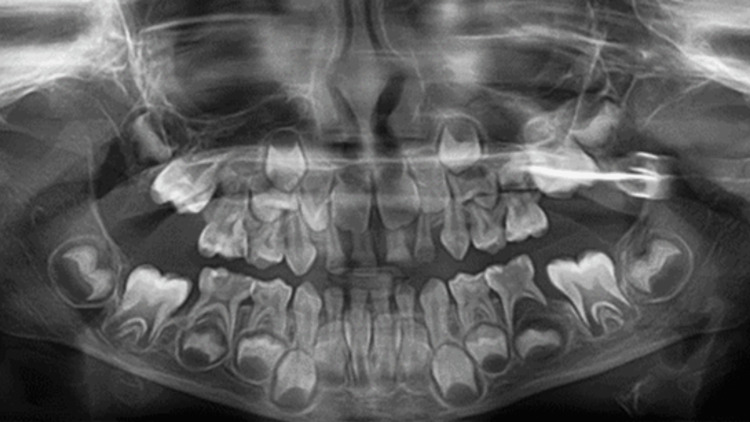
Preoperative panoramic radiograph showing generalized early childhood caries, teeth with advanced pulpal pathology, and radiographic signs of chronic oral infection associated with the second left lower primary molar.
